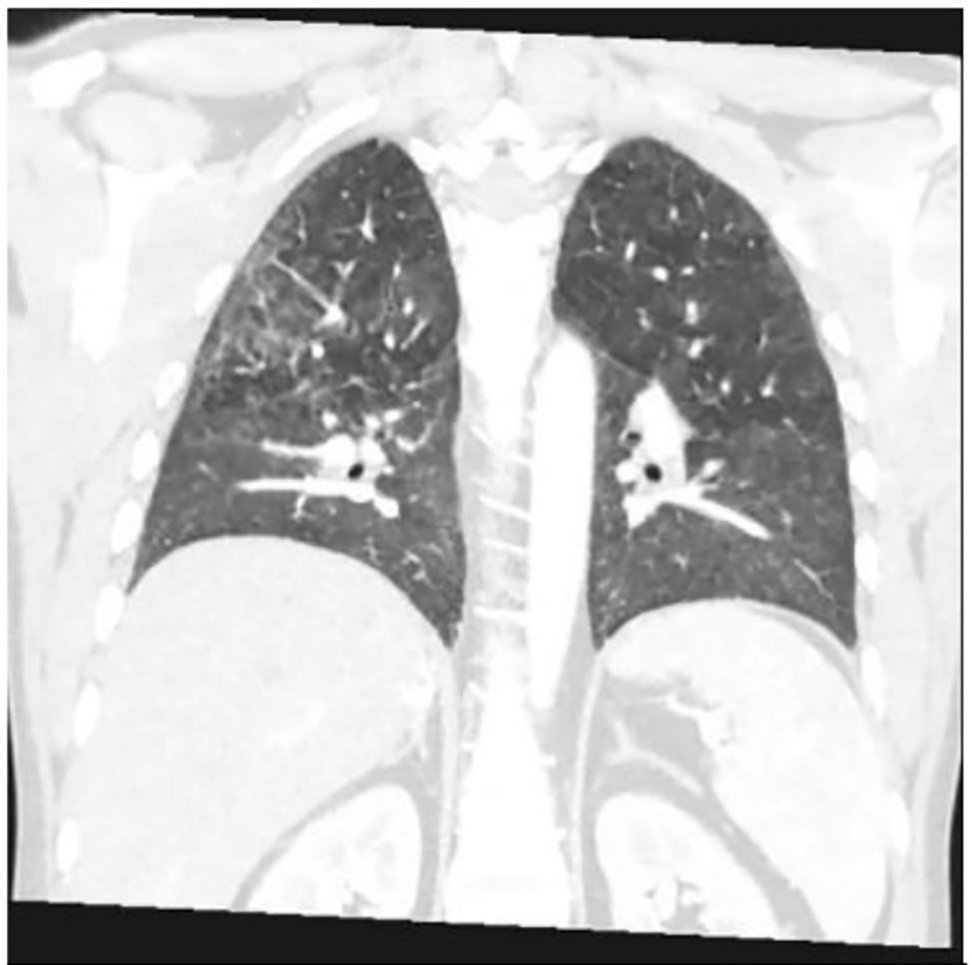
Coronal CT image obtained 3 months later with resolution of majority of the nodular opacities with residual ground glass opacities in right upper lobe
